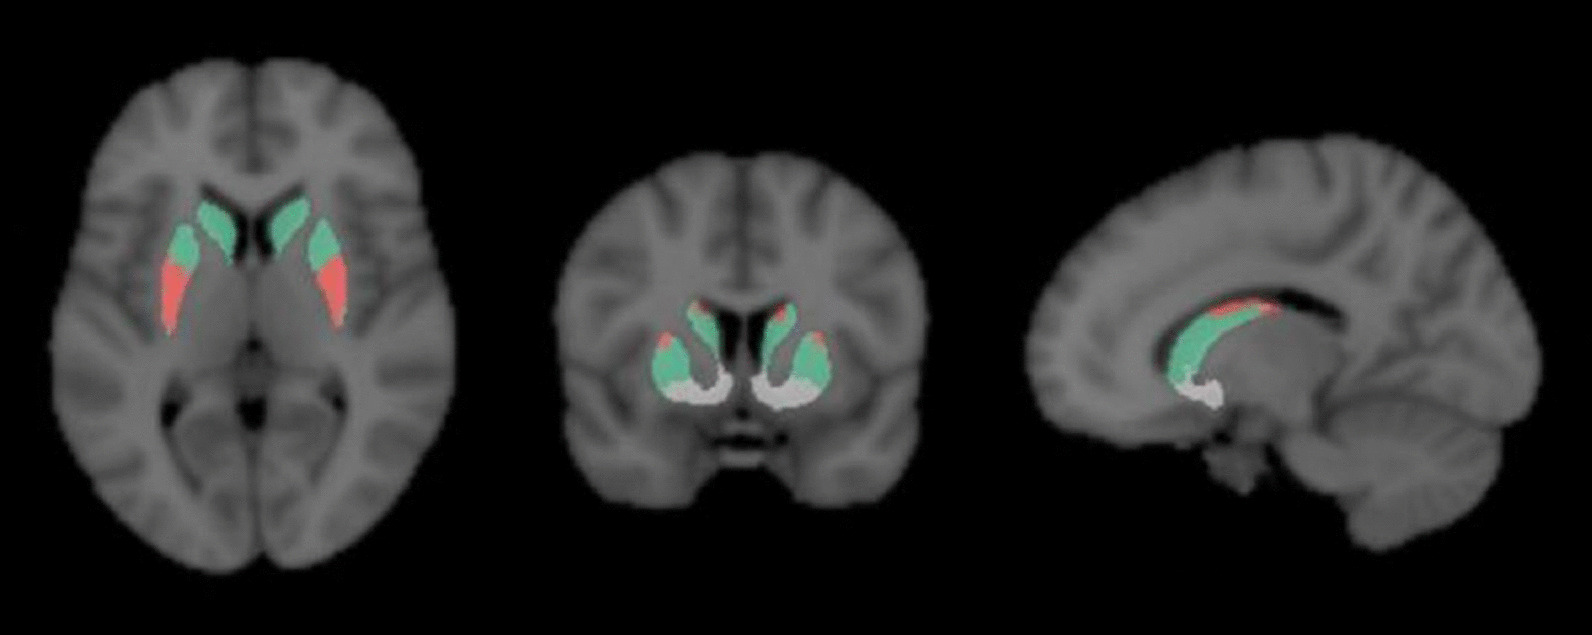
3D Functional subdivisions of striatum using the FSL-template overlaid on T1-weighted MRI sequences, with the sensorimotor striatum displayed in red. Figure previously published as Fig. 1 in Kerstens et al., Clin Transl Imaging 2020. Republication permitted under CC BY licensing
